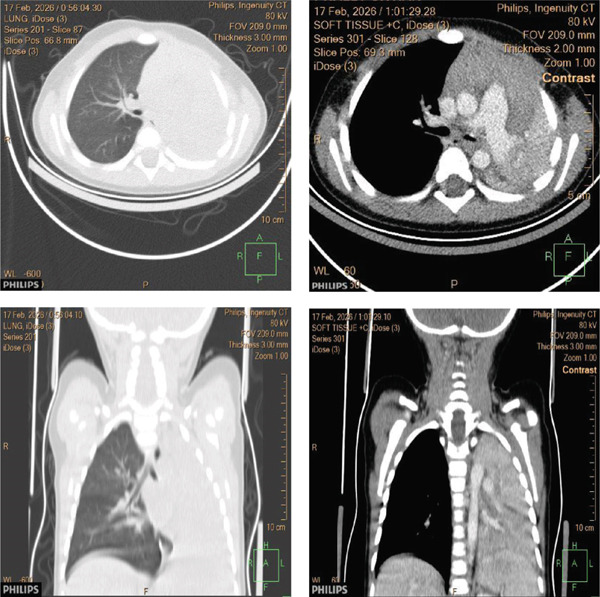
Contrast‐enhanced chest CT scan demonstrating complete left lung atelectasis and obstruction of the left main bronchus without evidence of pleural effusion or foreign body.
